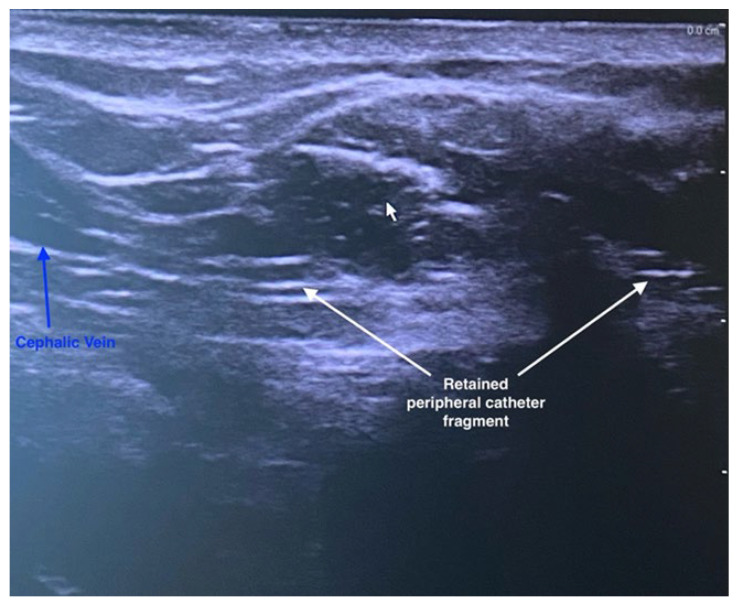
Postoperative ultrasound evaluation, after the first surgical exploration revealed the presence of a retained peripheral venous catheter fragment in the lumen of the cephalic vein, in the deltopectoral groove.
